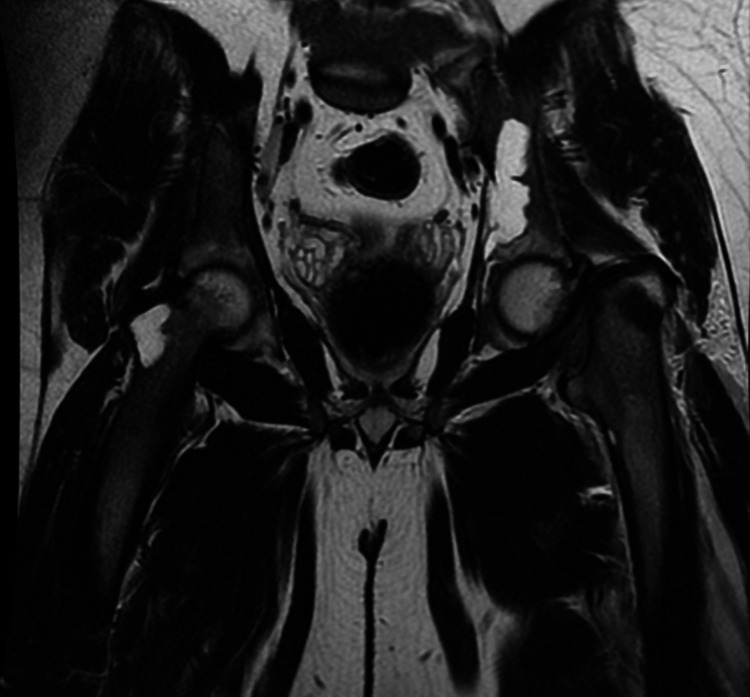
MRI hip: coronal T2 view showing multiple cystic lesions in the left acetabulum and right neck of femur region
